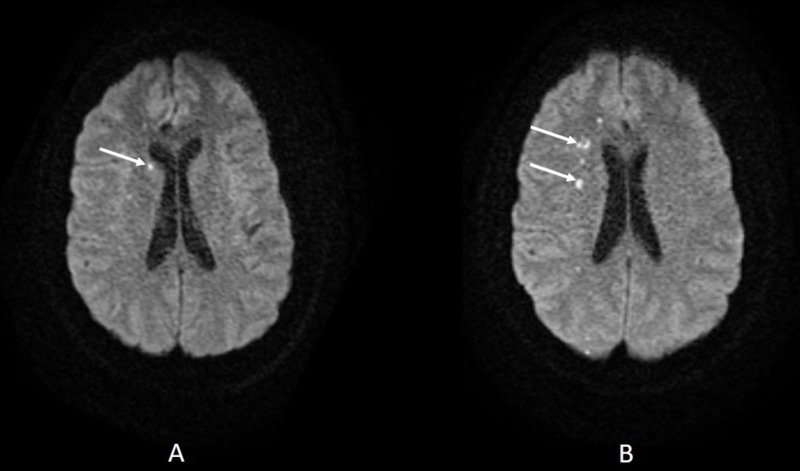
Diffusion-weighted magnetic resonance imaging of the brain showing punctate infarcts (white arrows).
